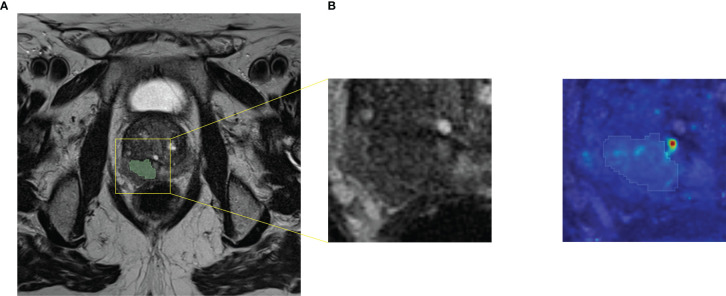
Axial T2w MR image of a PCa lesion with PI-RADS=5 and GS=4+5. (A) Original image together with its cropped version of size 64x64. We fed AG DL frameworks with the cropped images, i.e. the C-DS images. (B) Attention map obtained from the best performing AG DL framework fed with T2w C-DS images, superimposed to the original cropped version of the T2w image and its segmentation.
